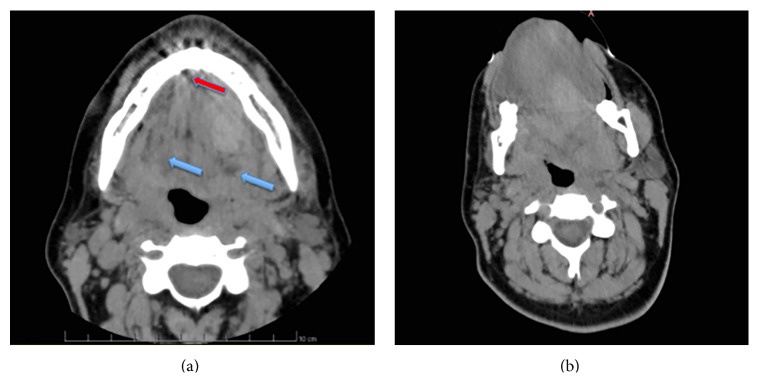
(a) Axial computed tomography image showing multiple areas of the whole mouth floor with different density (blue arrows) and the region of the cortical bone perforation (red arrow). (b) Axial computed tomography image showing the lingual, sublingual, and parapharyngeal haematoma with tongue displacement and restriction of the upper airways spaces.
